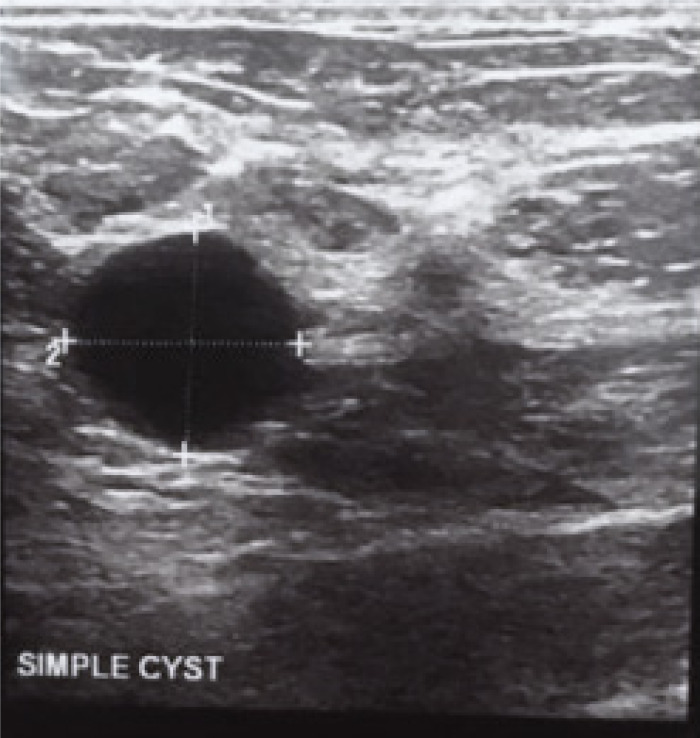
Simple cyst A 26-year-old female who complained of a painless lump in her right breast Ultrasound scan showing an anechoic mass with thin perceptible wall, through transmission and posterior acoustic enhancement Diagnosis: simple cyst
